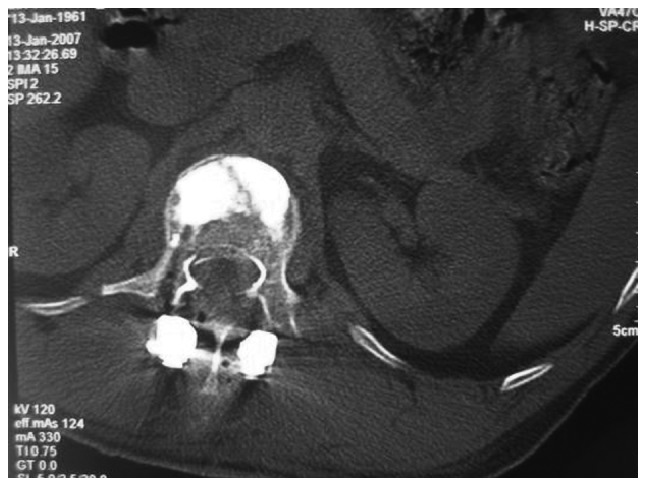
Postoperative CT scanning image of a 45-year-old male.
